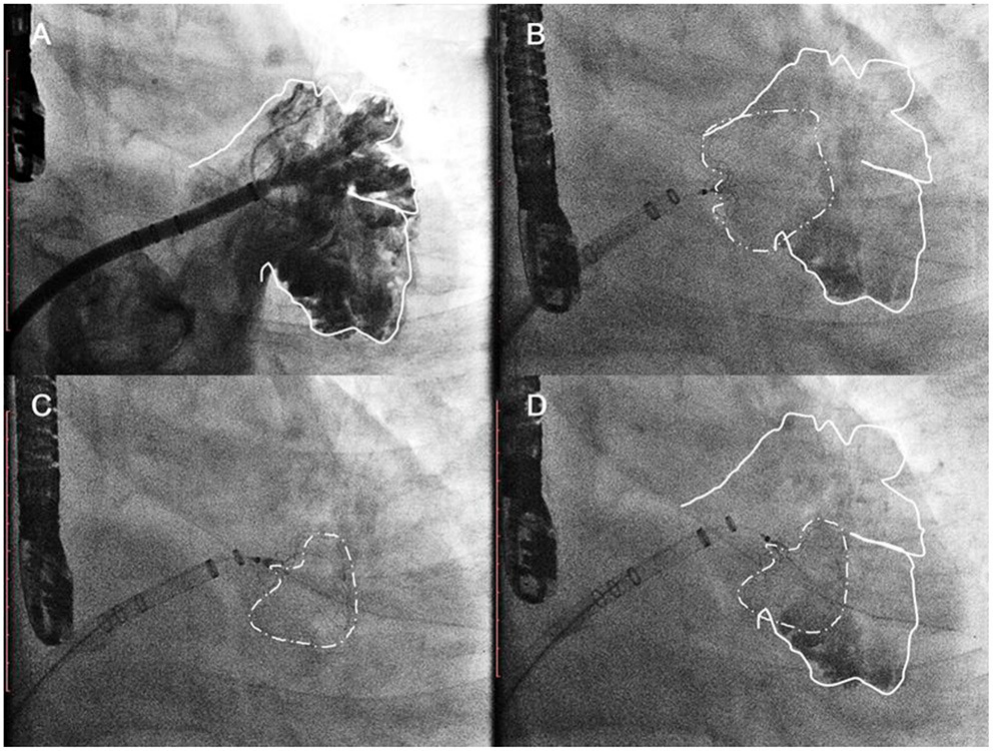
The common implantation strategy sequence. Step 1 (A) initial angiographic injection at right anterior oblique (RAO) 30°Caudal 20; Step 2 (B) a 33-mm Watchman device was initially chosen as the first attempt. Acquiring detail of the LAA anatomy through the TEE/CTA images was necessary before the dual-device strategy was selected. Step 3 (C) In the first implantation step, we chose an appropriately sized device to close the more challenging lobe of the LAA. Step 3 (D) The tug test and TEE were used to evaluate the PASS criteria with the first device.
